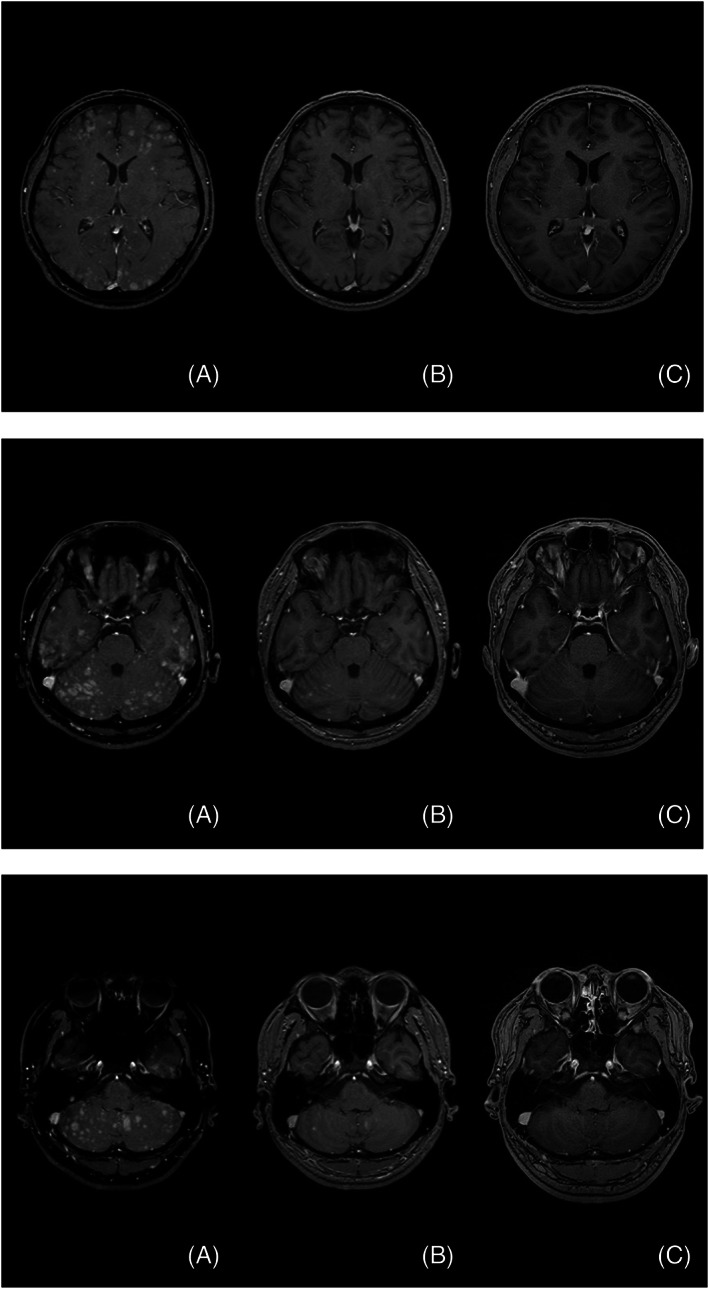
Contrast‐enhanced magnetic resonance imaging (MRI) scan of the head. Before lorlatinib administration, multiple brain metastases were observed (A); however, 12 days after lorlatinib administration, many of the metastases had disappeared (B), and after three months, multiple brain metastases were no longer detected on MRI (C).
